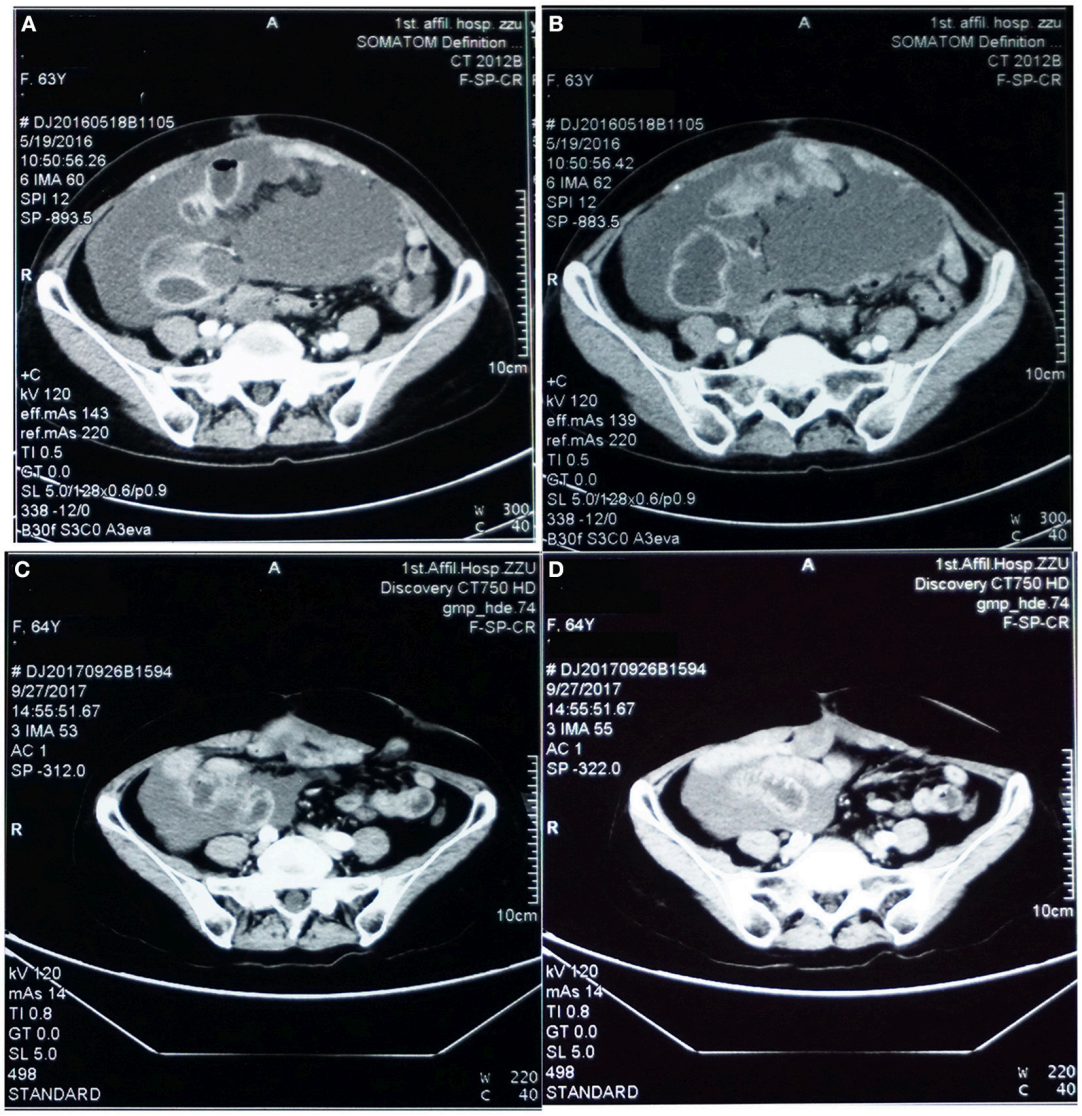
Pelvic CT images before (A,B) vs. after (C,D) targeted therapy. (A,B) Prior to targeted therapy, images showed intraperitoneal multiple nodules, and a large number of peritoneal cavities filled up with mucus. (C,D) After targeted therapy using bevacizumab and oxaliplatin, image on the same sections showed significantly reduced peritoneal nodules and mucous cavities, suggesting stabilization of disease progression and partial response.
